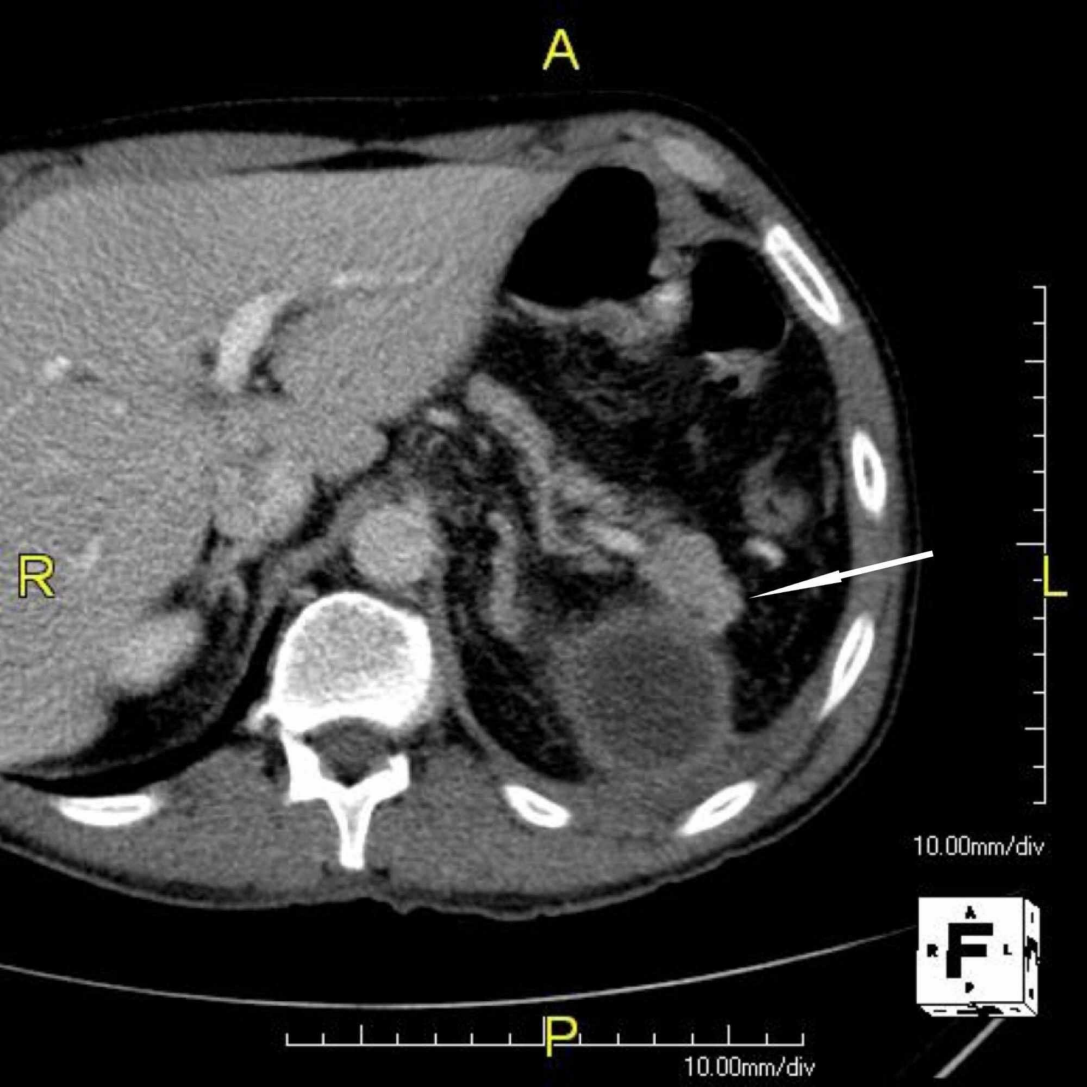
Contrast-enhanced axial CT image obtained during portal venous phase The main finding of the postsplenectomy pancreatic pseudocyst. The presence of an intrapancreatic accessory spleen (arrow) can be speculated in the tail of the pancreas CT: computed tomography
